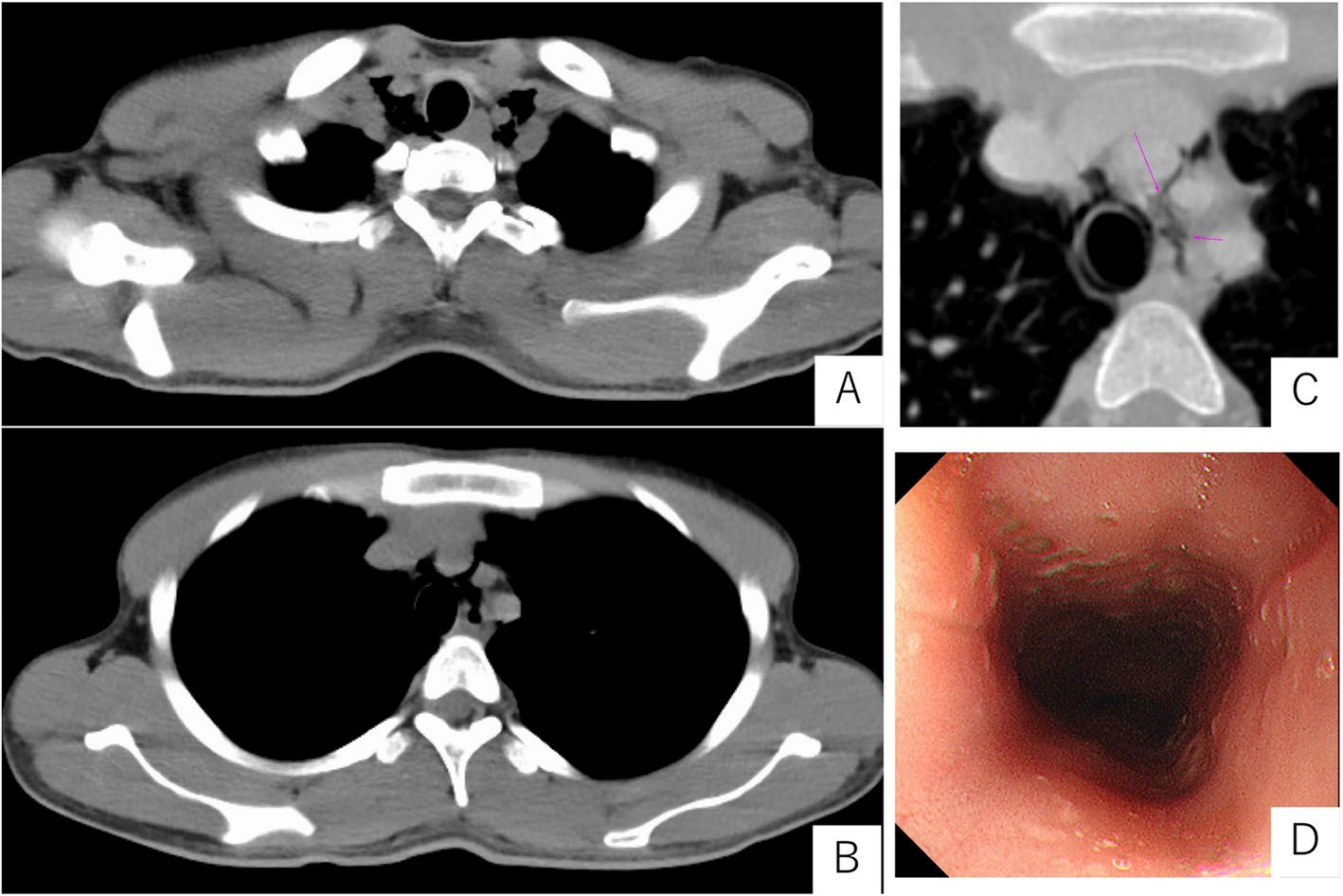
Computed tomography (A–C) and an image of upper gastrointestinal endoscopy after admission to our hospital
